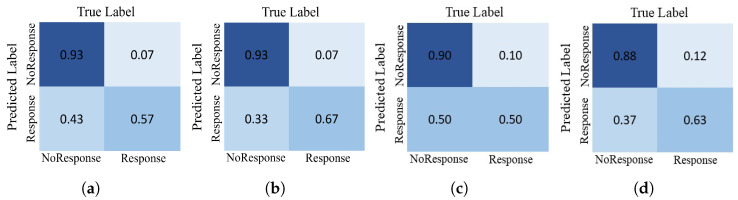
Confusion matrices of the proposed model for different inputs: (a) only T1 MRI scans; (b) $T_{1}$ MRI scans with clinical information; (c) $T_{1}$ and $T_{2}$ MRI scans with clinical information; and (d) $T_{1}$, $T_{2}$, and $T_{3}$ MRI scans with clinical information, over ISPY-1 dataset.
